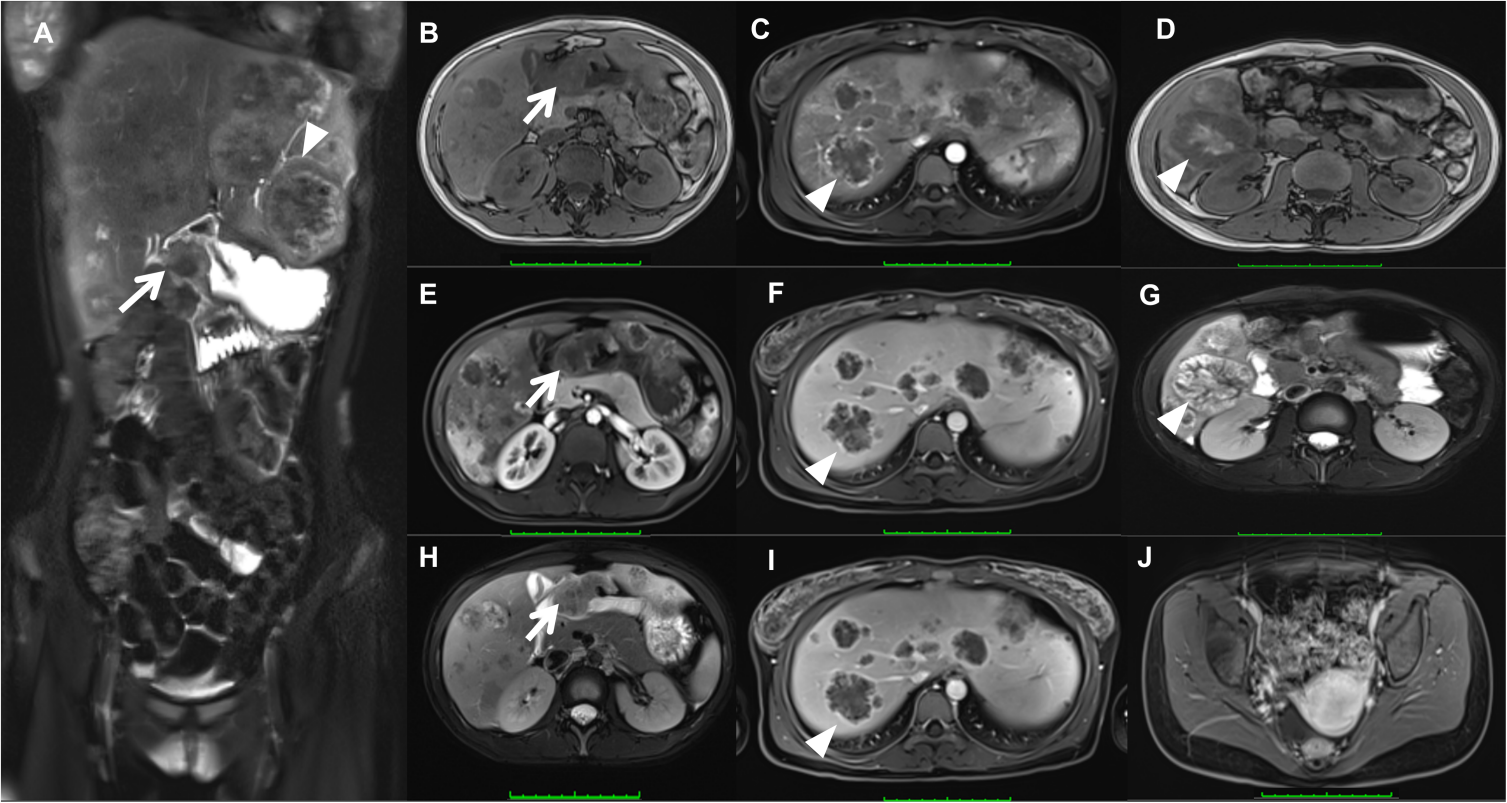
Magnetic resonance imaging (MRI) showed heterogeneous thickened of the gastric sinus wall (arrow) on T1WI (B), T2WI (A, H), and DEC-MRI (E); Multiple intrahepatic masses (triangles) demonstrated on T1WI (D), T2WI (A, G), and DEC-MRI (arteria phase, (C); portal venous phase, (F); delayed phase, (I). No apparent lesion was discovered in the uterus (J).
